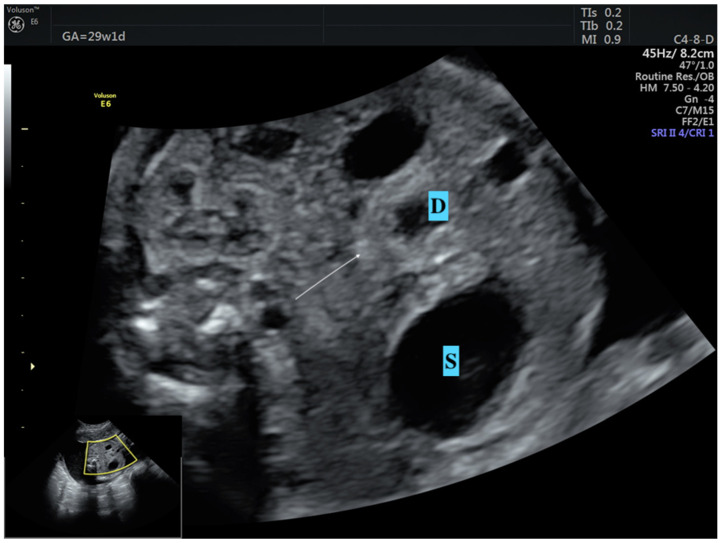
Prenatal sonographic appearance of annular pancreas at 30 weeks of gestation. Hyperechogenic band (arrow) surrounding duodenum (pliers sign) is the major finding of annular pancreas. S: Stomach, D: Duodenum.
